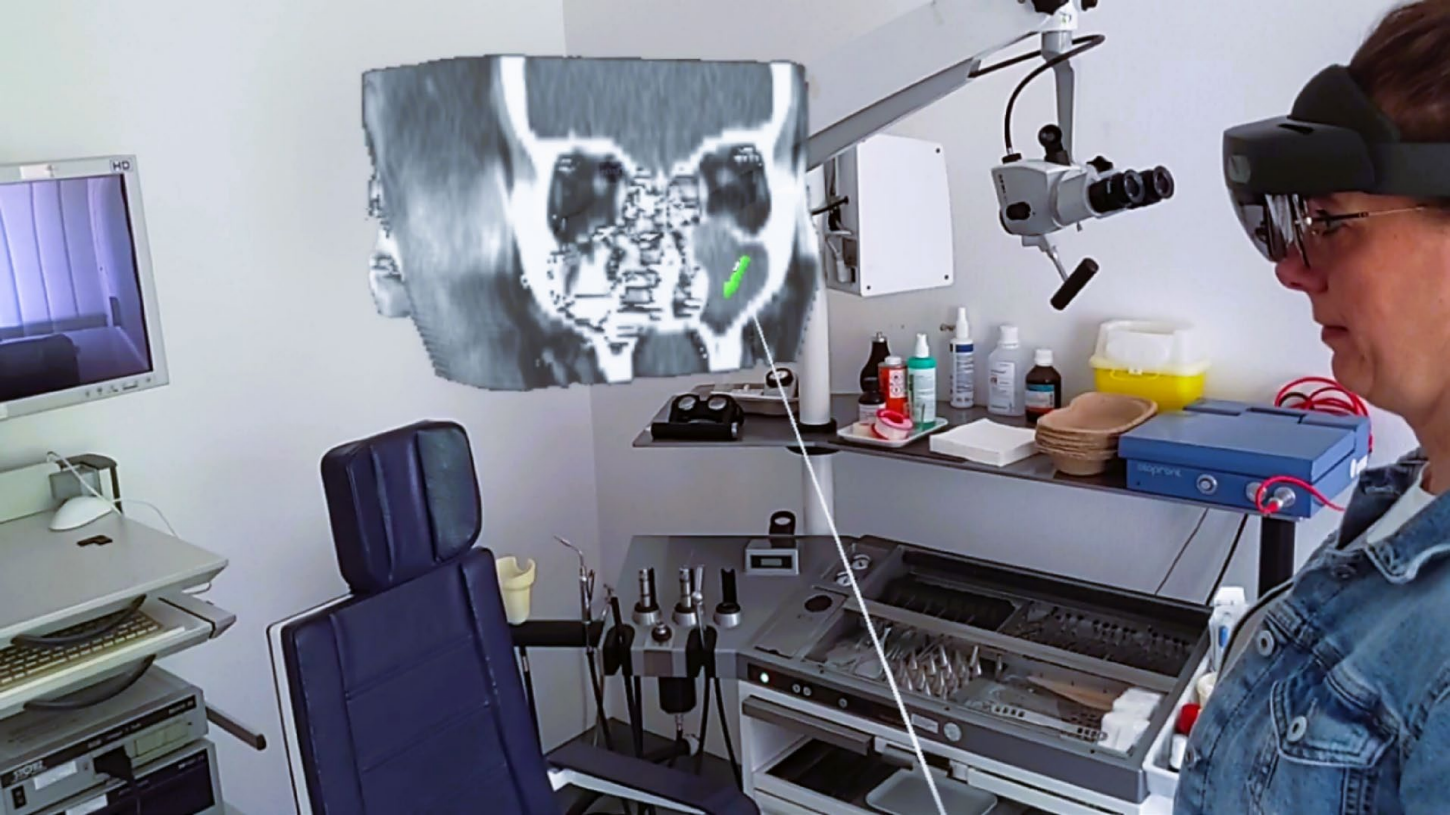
Real educational situation from the perspective of the educator. Both the educator and the patient look at the patient’s own CT scan of the skull as a 3D hologram. Written informed consent was obtained by the participant to publish the image.
